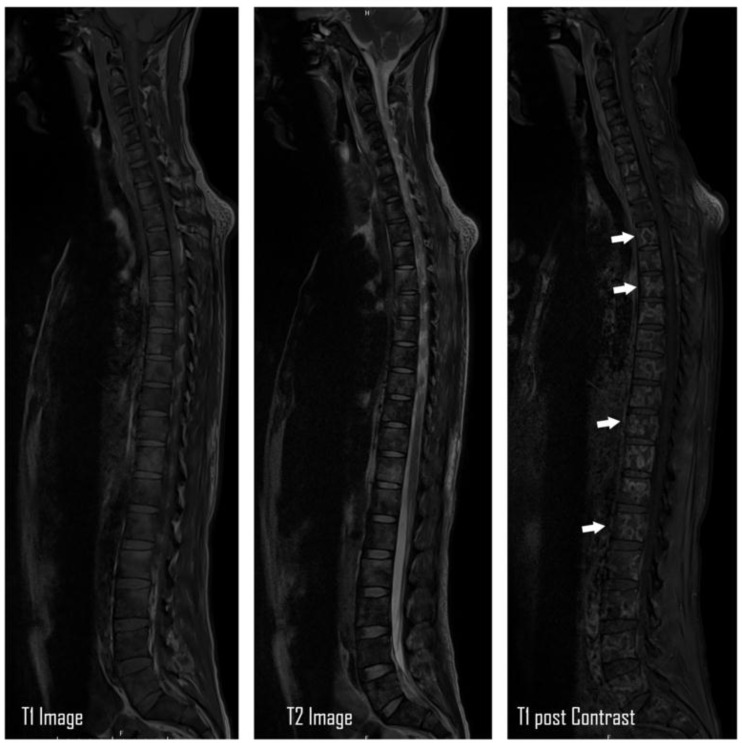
MRI spine showing multiple lytic lesions demonstrating post-contrast enhancement (widespread variable-sized bony metastatic versus myeloma lesions in the cervical, thoracic, lumbar, and sacral spine). Lesions exemplified with solid white arrows.
